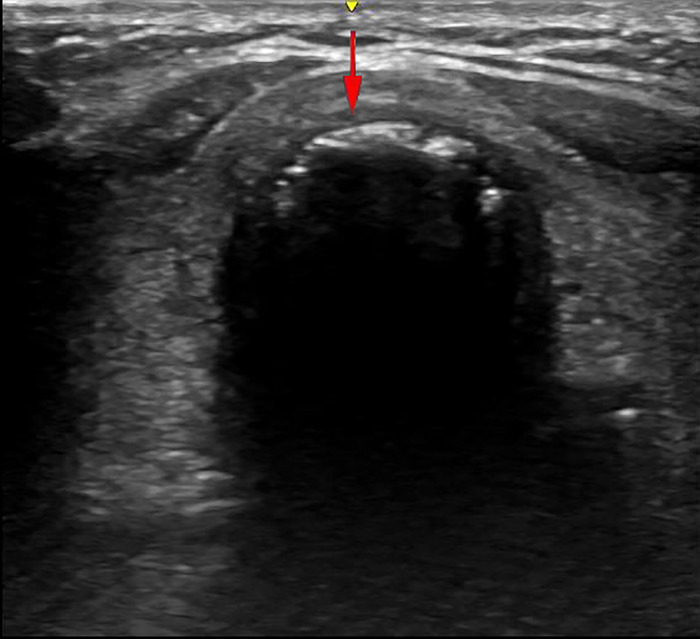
The inter-annular space between the second and third tracheal ring. The yellow and red arrows are the indication marks of the ultrasound probe and the direction of the puncture needle, respectively.
